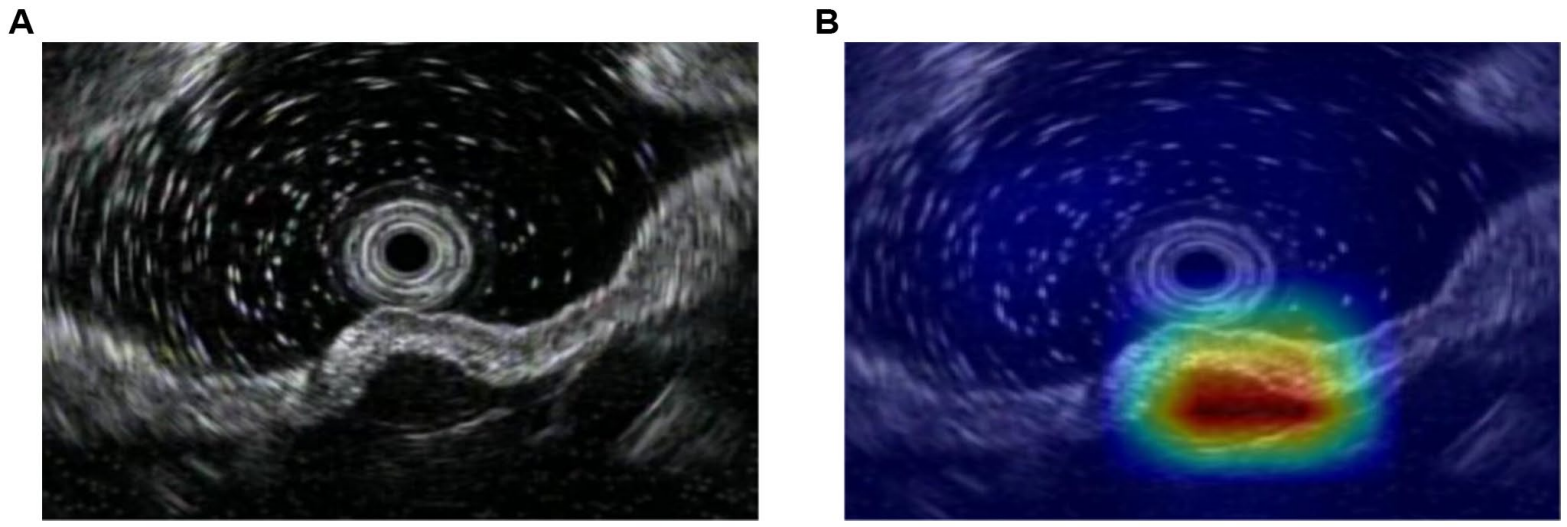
Visualization of model interpretability. (A) A representative original endoscopic ultrasonography (EUS) image showing a gastric subepithelial lesion. (B) The corresponding feature activation heatmap generated by the MedMamba model. Warmer colors (red) indicate regions with higher influence on the model’s classification decision, demonstrating that the model accurately focuses on the target lesion rather than surrounding artifacts or healthy tissue. (A) Original image, (B) Heatmap of the leision.
